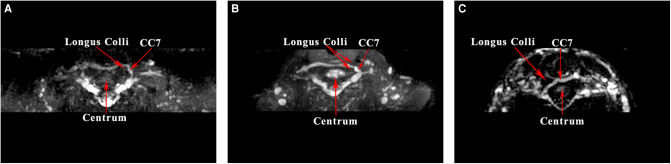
contralateral cervical 7 (CC7) nerve transfer operation on MRI by 3D CUBE-STIR sequences: (A) anterior route, (B) middle route, (C) posterior route.
